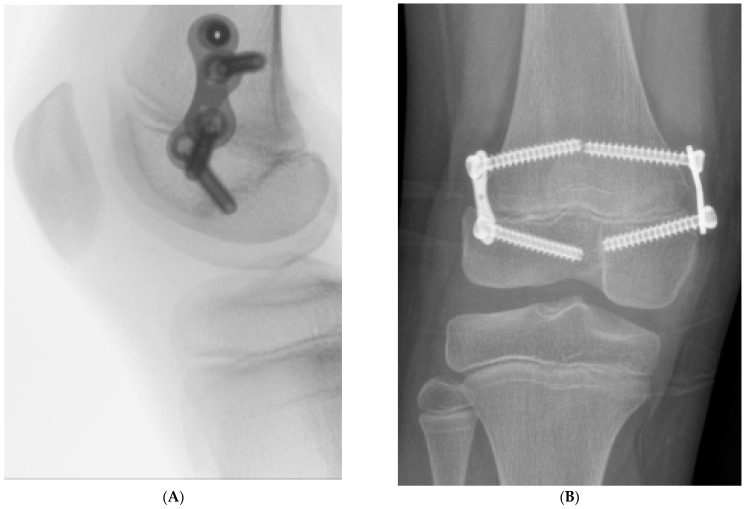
Radiographs showing the 8-plates in a horizontal orientation, with (A) representing the lateral view and (B) representing the anteroposterior (AP) view, indicating the end of their correction potential in the RGG process.
